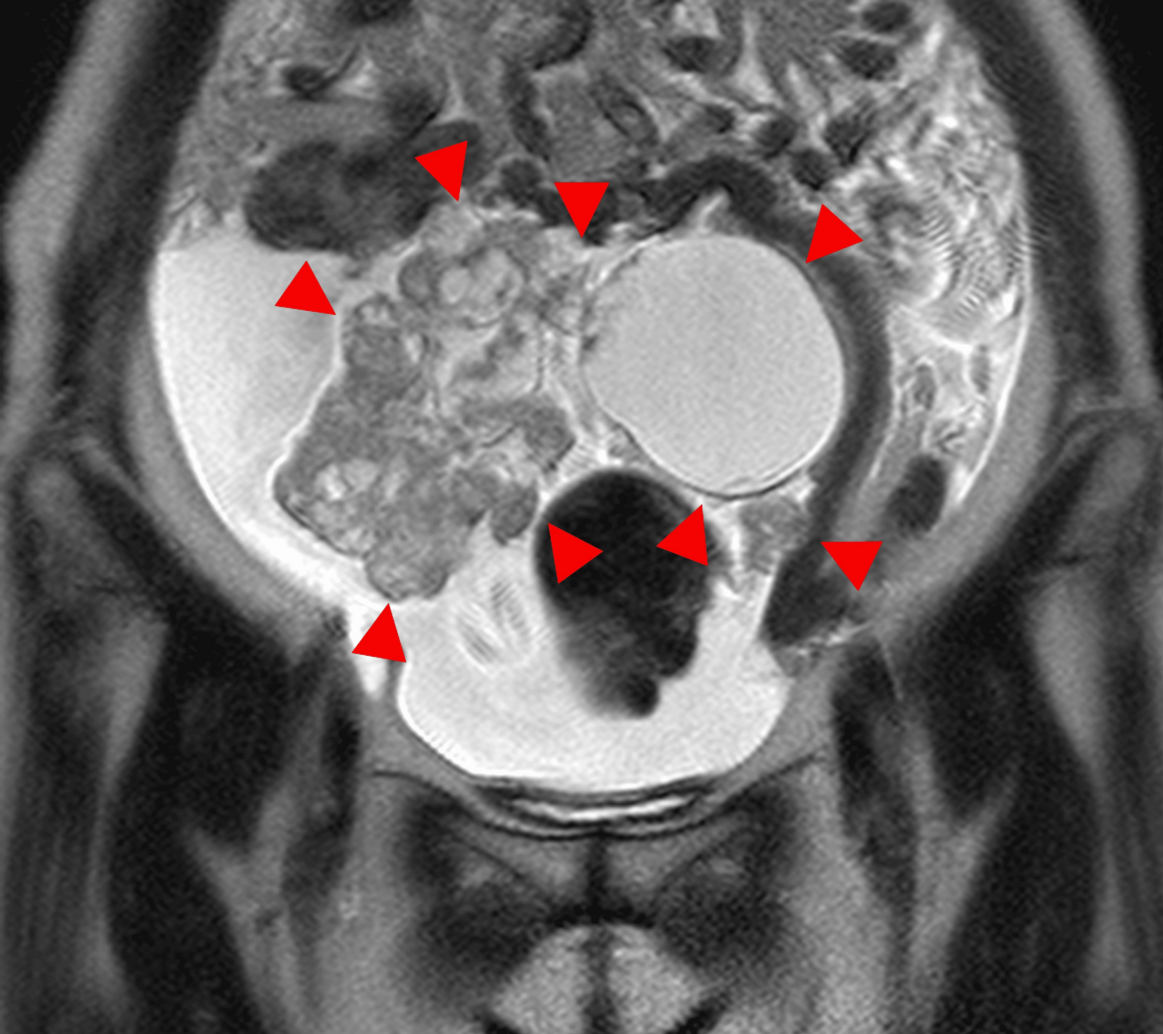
MRI of ovarian carcinosarcoma in a 61-year-old woman with a 17-cm tumor in the right ovary The coronal T2-weighted image shows a mass with an irregular margin, associated ascites, solid components, and cystic areas (arrowhead). The solid component demonstrates heterogeneous signal intensity.
